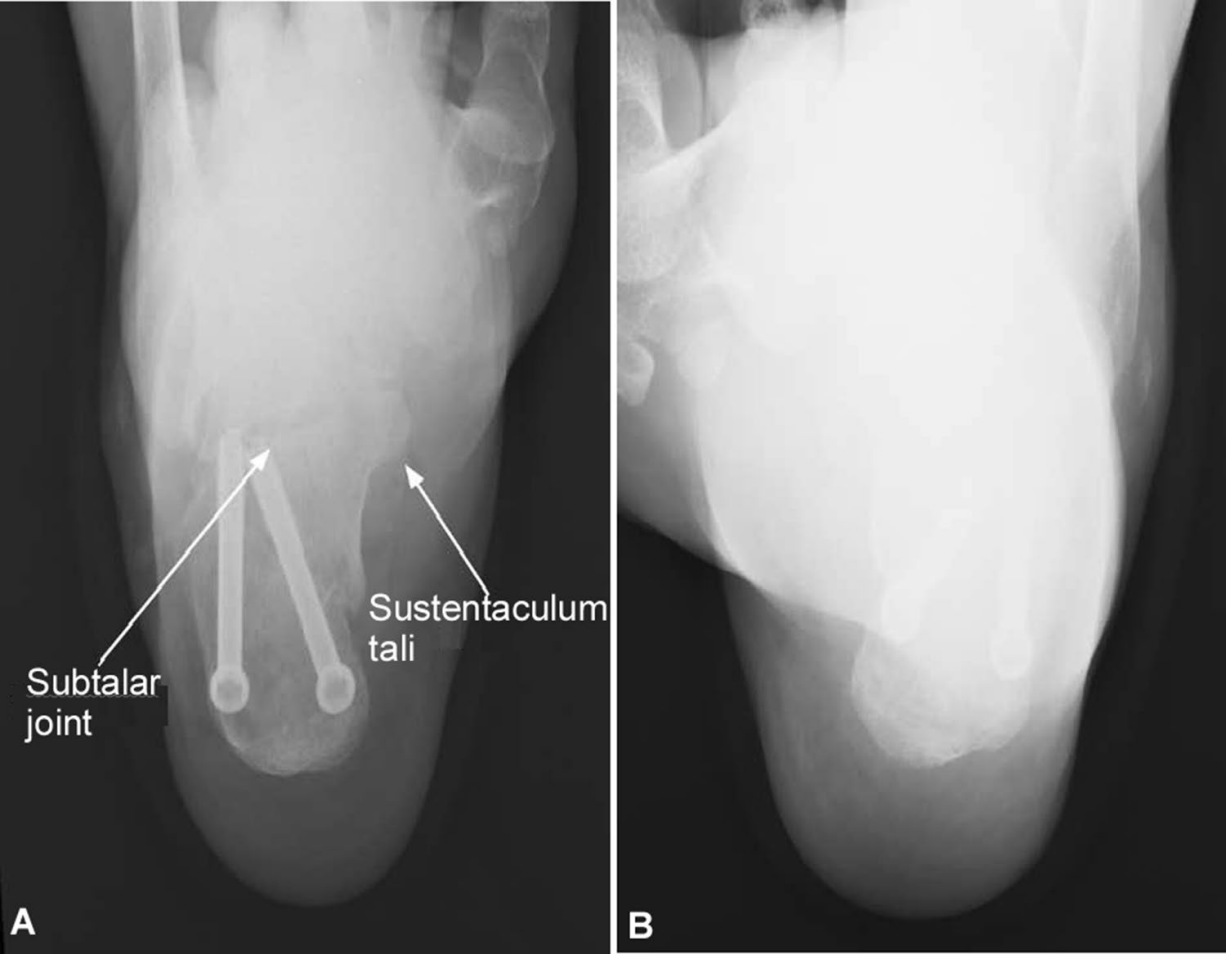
A male patient (30 years of age) had internal fixation for calcaneal fracture. (A) Modified horizontal calcaneal axial radiograph can clearly show the subtalar joint and sustentaculum tali. The calcaneus is without deformation. (B) Regular calcaneal axial radiograph cannot clearly demonstrate the subtalar joint or the sustentaculum tali.
